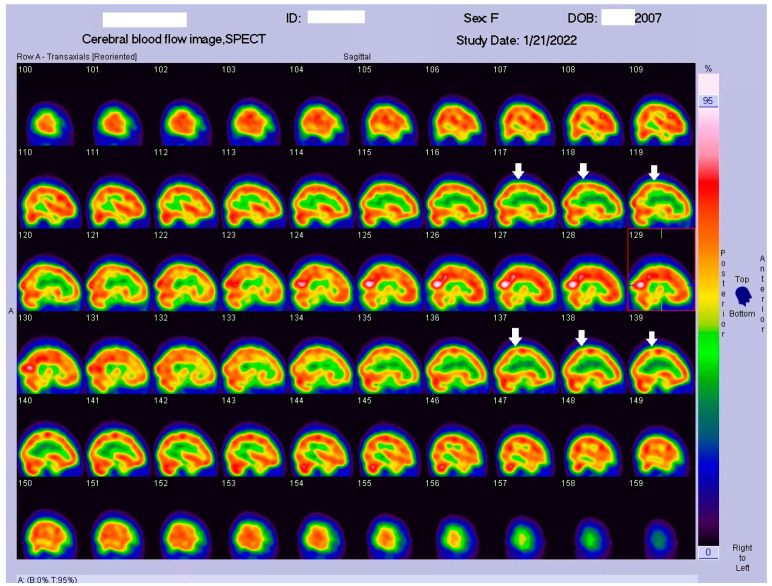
Sagittal view of the Tc−99m ECD brain perfusion SPECT images. A decreased uptake in the tracer can be observed in the parietal area, especially in the postcentral gyrus (arrow).
